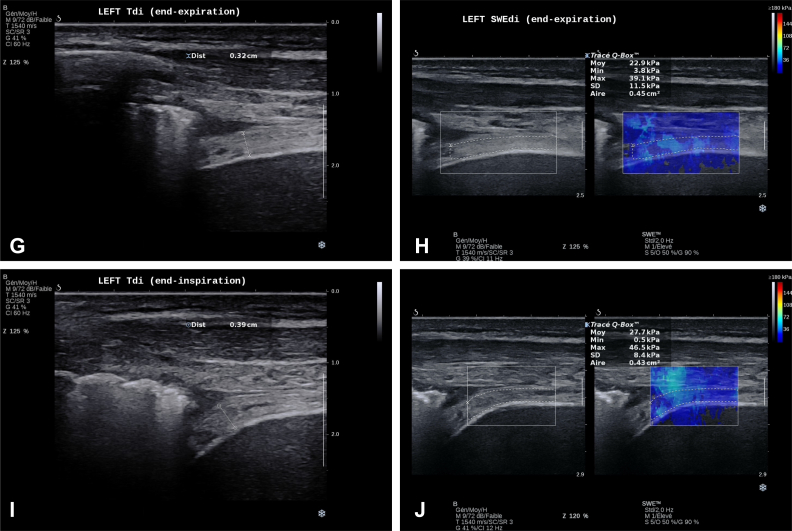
A-J, Left diaphragm at both time points of assessment. A, Left Tdi at end-expiration during high-PS (18 cmH_2_O): first time point of DUS assessment. White arrows indicate regions of diaphragmatic tissue injury. B, Left SWEdi at end-expiration during high-PS (18 cmH_2_O): first time point of DUS assessment. C, Left Tdi at end-inspiration during high PS (18 cmH_2_O): first time point of DUS assessment. D, Left SWEdi at end-inspiration during high-PS (18 cmH_2_O): first time point of DUS assessment. E, Left Tdi at end-expiration during low-PS (8 cmH_2_O): first time point of DUS assessment. F, Left SWEdi at end-expiration during low-PS (8 cmH_2_O): first time point of DUS assessment. G, Left Tdi at end-expiration: second time point of DUS assessment. H, Left SWEdi at end-expiration: second time point of DUS assessment. I, Left dTdi at end-inspiration: second time point of DUS assessment. J, Left SWEdi at end-inspiration: second time point of DUS assessment. Aire = area; Dist = distance; DUS = diaphragm ultrasound scan; Max = maximum; Min = minimum; Moy = mean; PEP = positive expiratory pressure; PS = pressure support; SWEdi = diaphragm shear-wave elastography; Tdi = diaphragm thickness.
